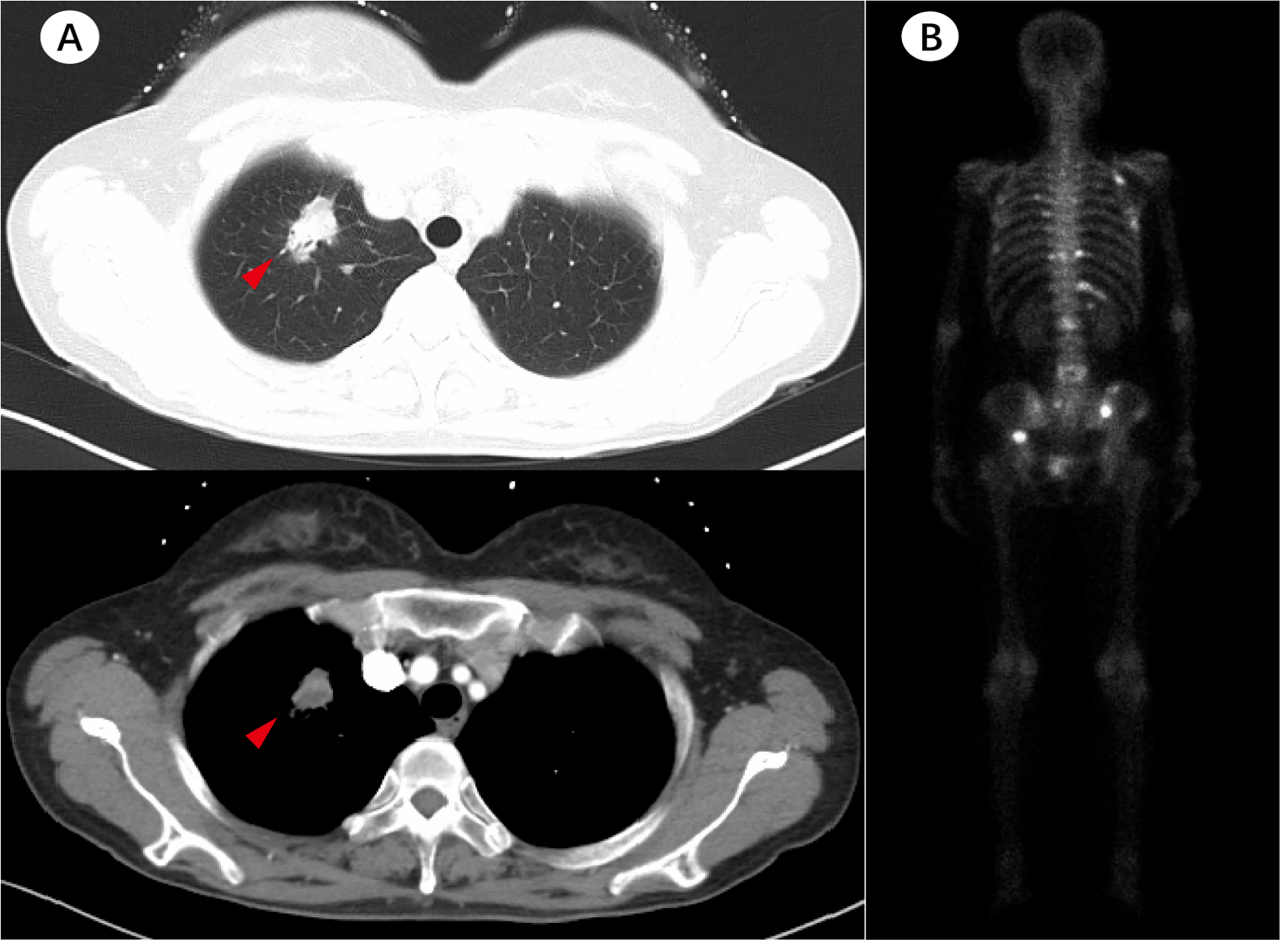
Patient’s imaging before and during anti-tumor treatment. (A) The baseline chest computed tomography (CT) scan before treatment. (B) Bone isotope scanning suggested extensive bone metastases after 12 months of Osimertinib treatment.
